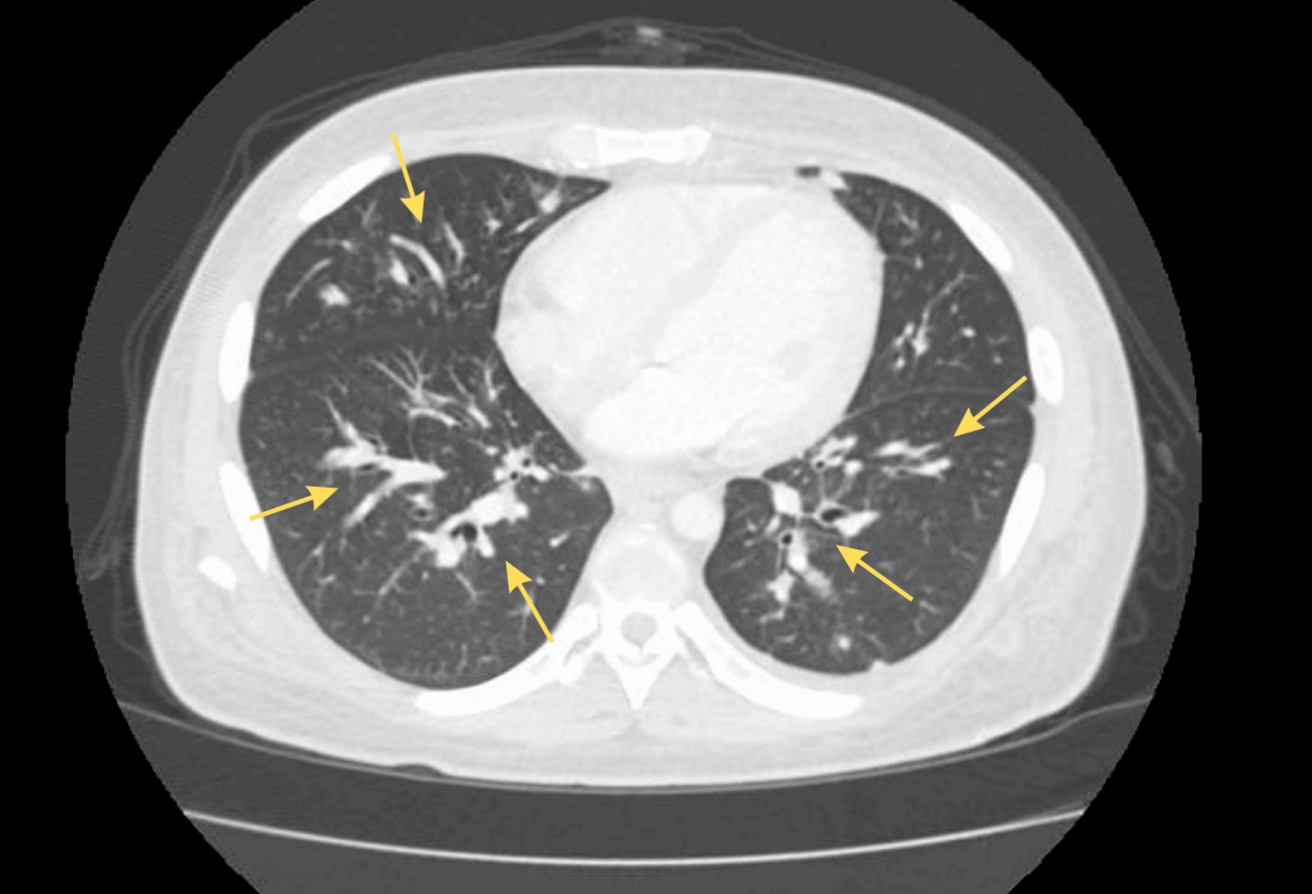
CT scan showing diffuse bilateral lung opacities with multiple nodular infiltrates (yellow arrows). CT: Computed tomography
